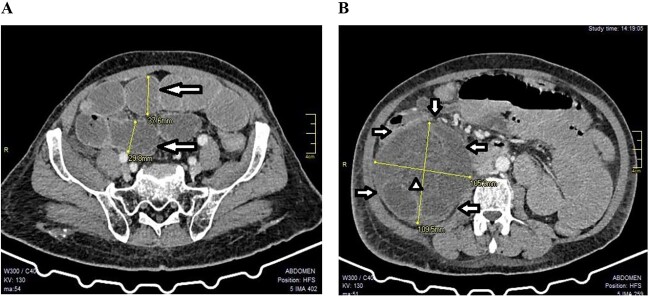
A CT scan showed features of intestinal obstruction around the paracecal site with dilated small bowel loops; (A) the various dilated small bowel loops (arrows) can be seen; (B) the hernia sac (arrows) with dilated bowel loops inside (arrow head) were seen around the cecum (axial images).
